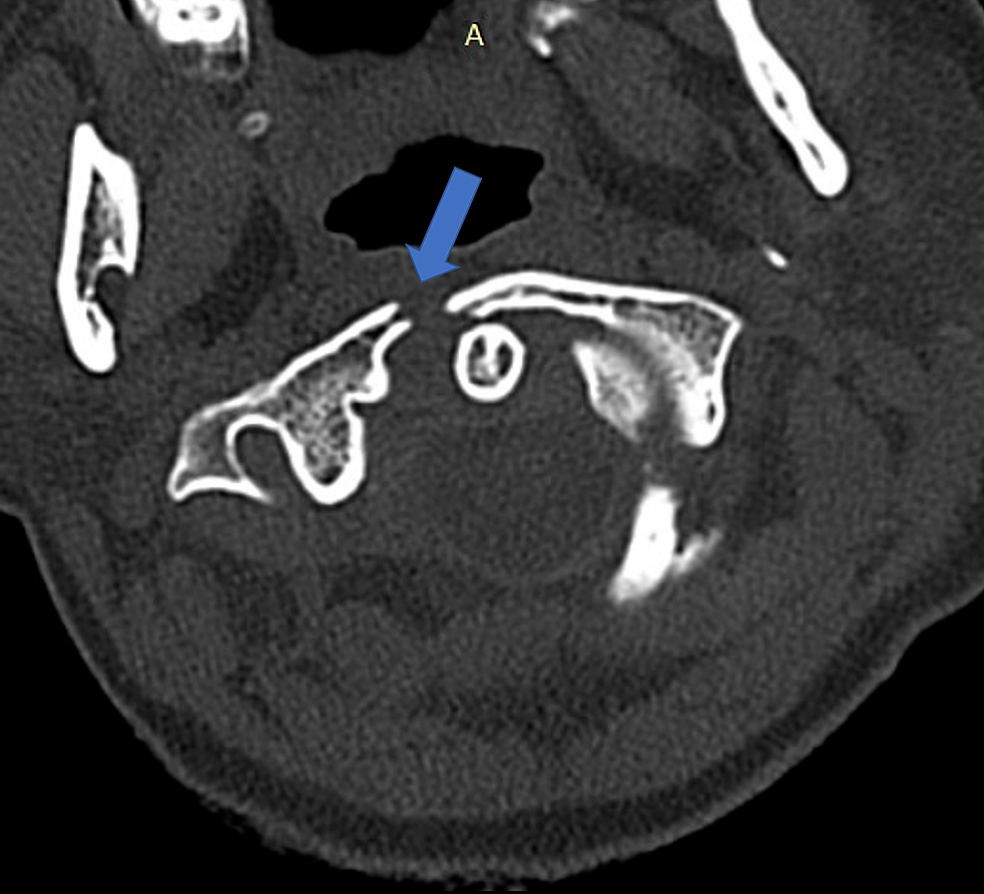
cervical CT axial view: increased angulation of the right C1 anterior arch fracture (blue arrow)
